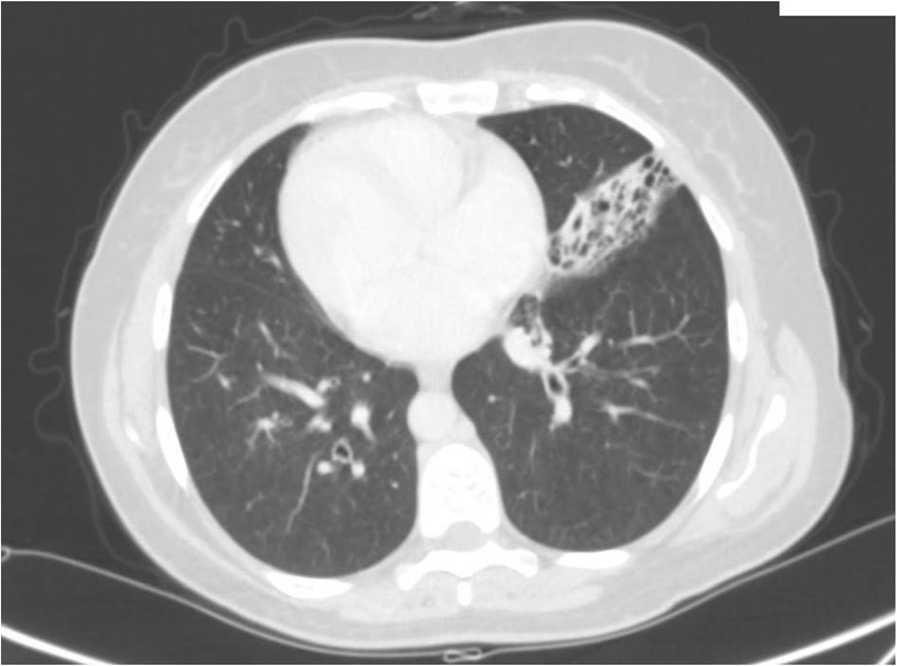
CT-scan performed in patient #4 during hospitalization for pneumonia in upper left lobe.
